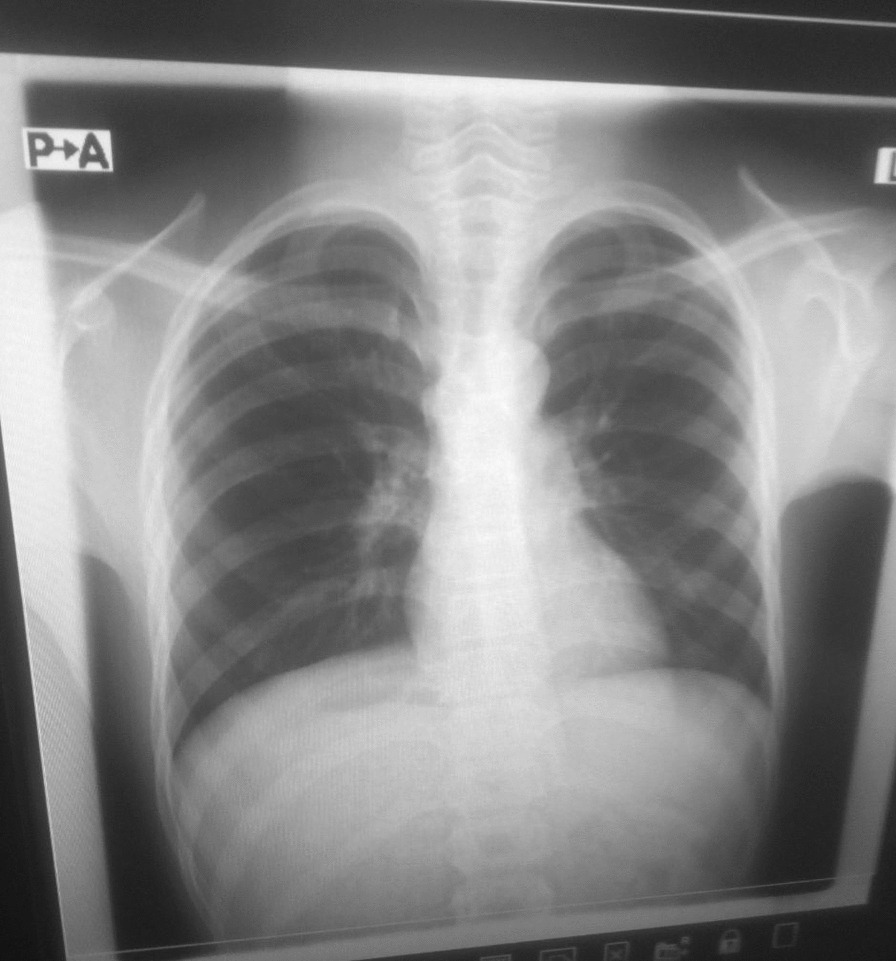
Posteroanterior chest x-ray showing cardiac apex positioned towards the left
